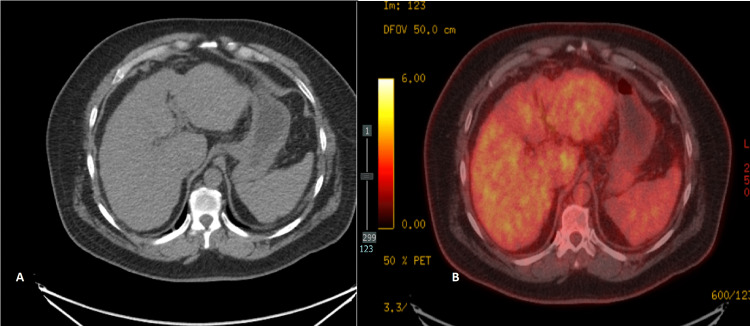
August 2022 PET/CT scan of the abdomen There was no radiological evidence of active hepatic disease in both A (CT scan) and B (PET scan) PET: Positron emission tomography
